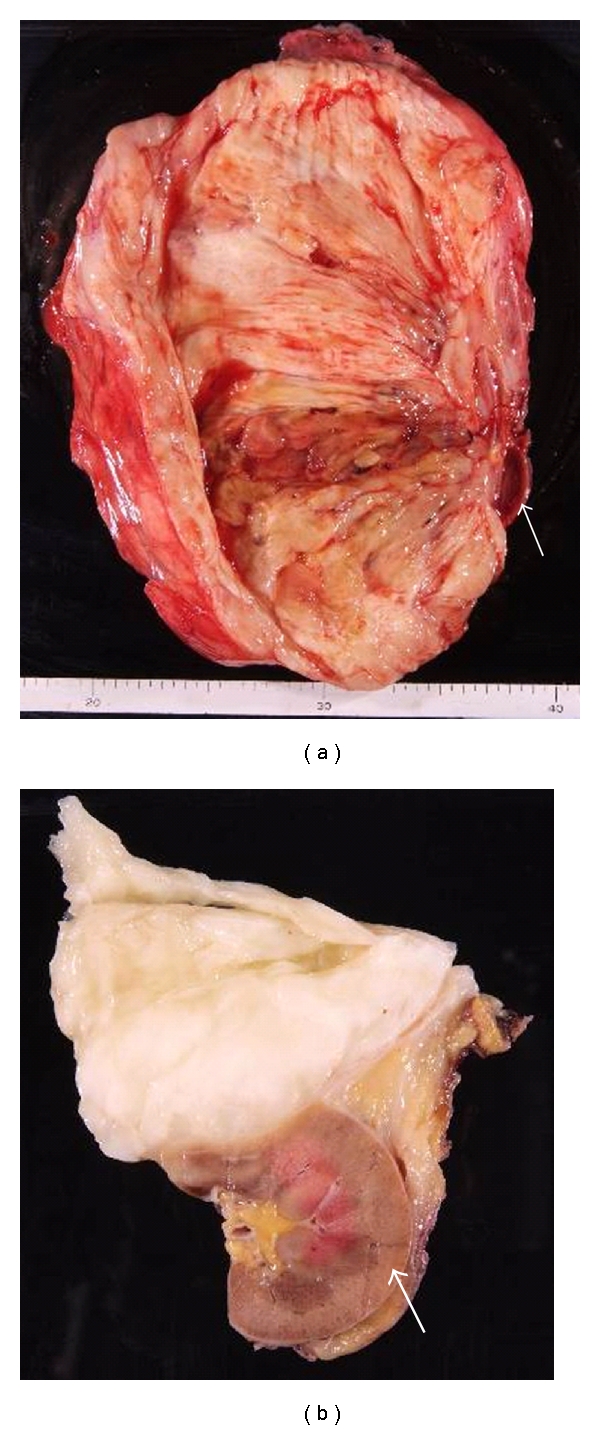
(a) shows resected mass and (b) shows two component of tumor as determined by CT and MRI after fixation. Macroscopically, the resected mass weight was 8.5 kg. The giant tumor was white, and the small one was yellowish (arrow: right kidney).
